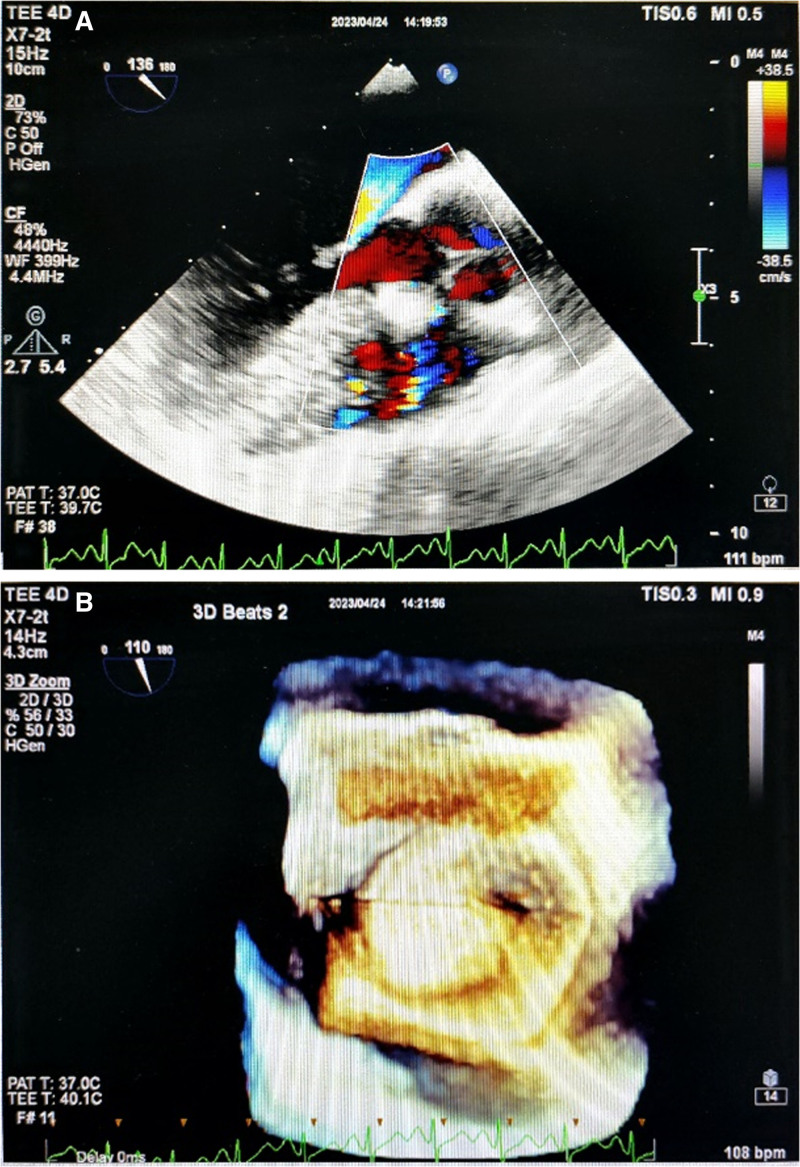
Post-procedure transesophageal 3-dimensional echocardiography (no significant shunt was observed at atrial and ventricular levels).
